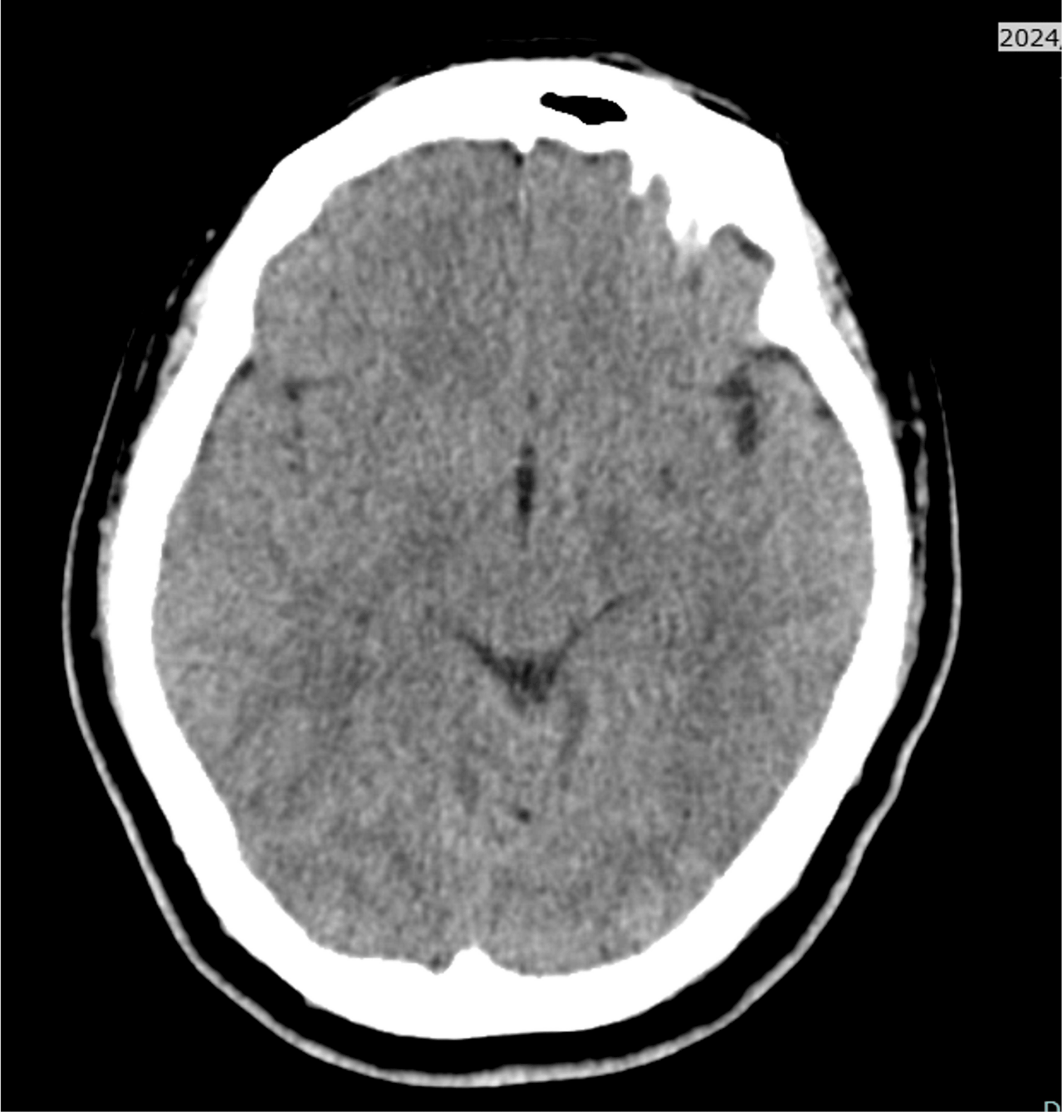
CT Head on postoperative day 4 Improved visualization of the basal cisterns was observed, suggesting resolution of intracranial hypertension.
